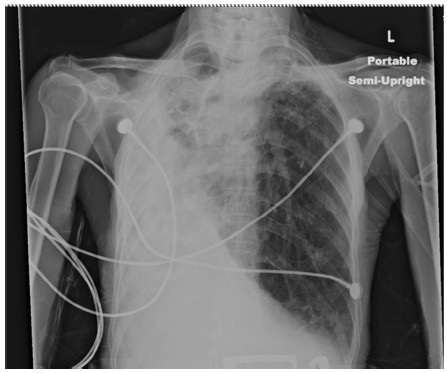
Chest radiograph showing gross mediastinal deviation toward the right lung and overexpansion of the left lung.
